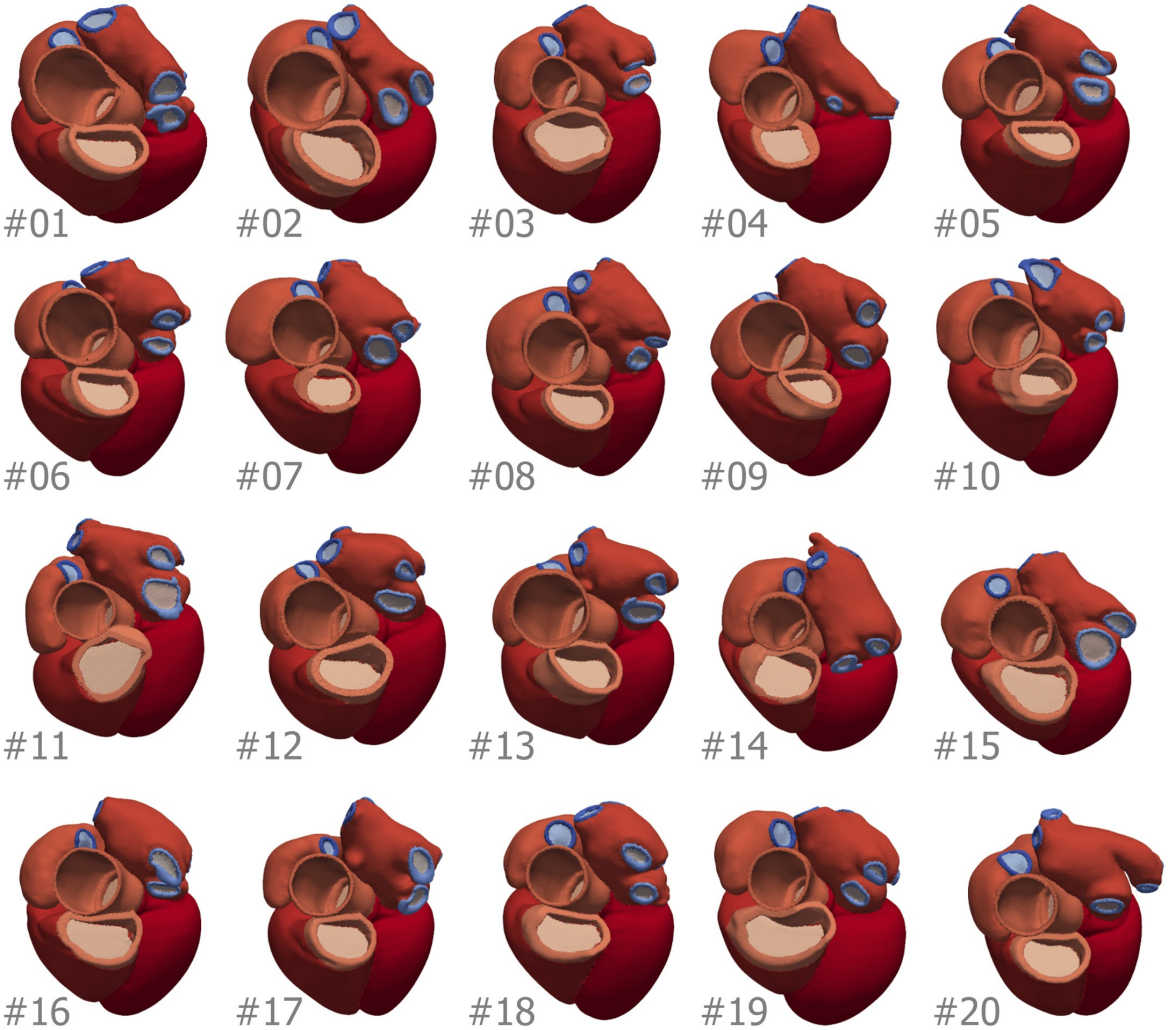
Meshes of the 20 cases of the CT cohort, with the number of the case in the bottom left corner of each image. Colour indicate mesh labels described in S1 Text and referenced in the tables in S5 Text. For the SSM construction we discarded mesh #20.
